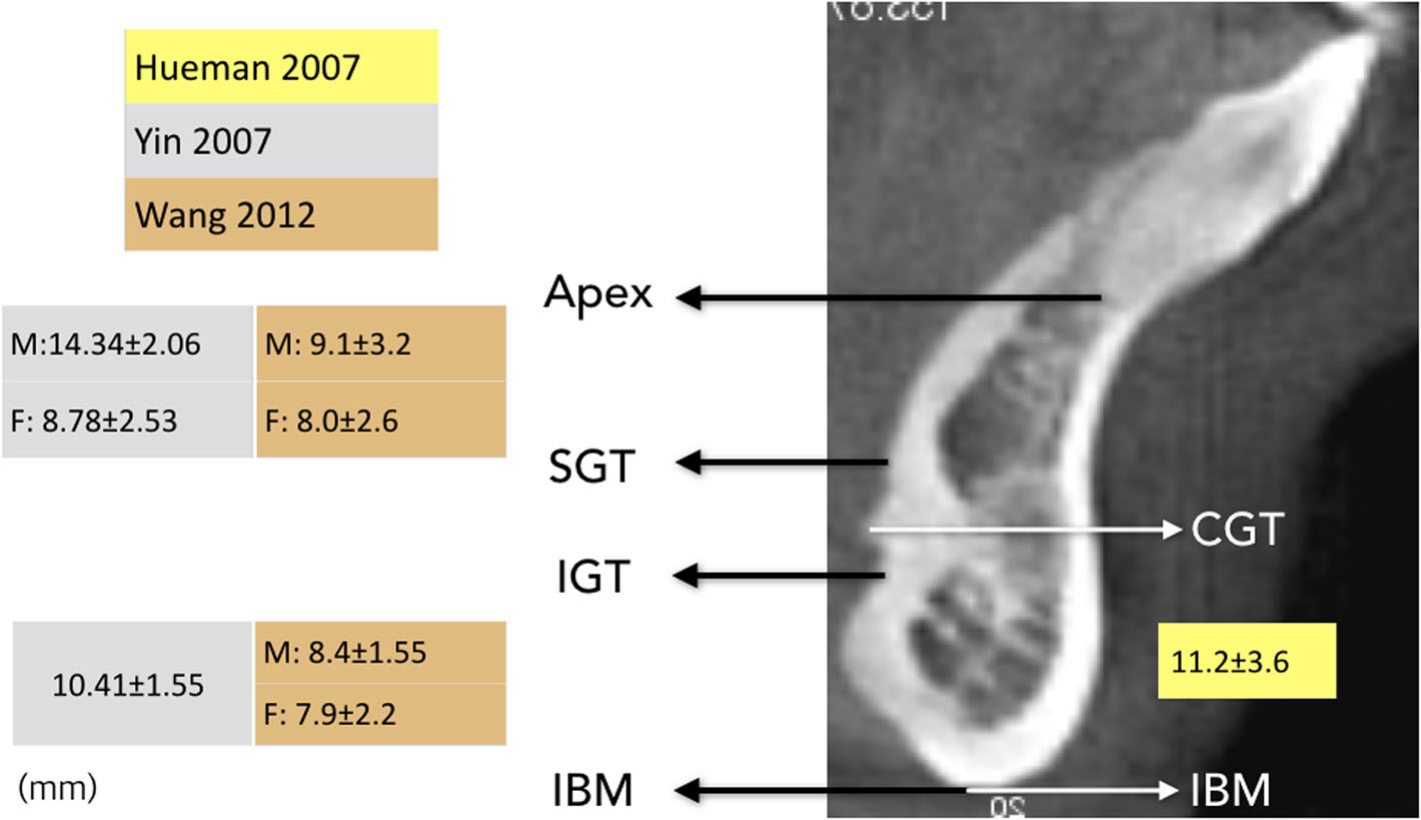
Position of the genial tubercle evaluated by radiographic measures
